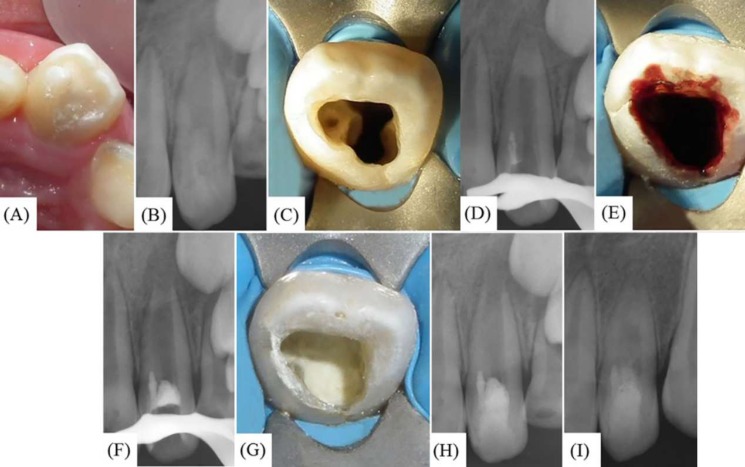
A)* Maxillary left lateral incisor exhibits a cone-shaped morphology; *B)* Pre-operative radiograph showing Oehlers type II DI; *C) *Access cavity depicting two canal orifice entrances; *D)* Radiographic verification of mineral trioxide aggregate (MTA) placement in the invagination; *E)* Blood clot formation; *F)* Radiographic verification of MTA placement in the main canal; *G)* Clinical photograph of white MTA placement in the main canal; *H)* Immediate postoperative radiograph of revascularization technique; *I)* 32 month follow-up with radiographic evidence of notable increase in the thickening of the apical radicular walls, an incrementing in the root length, and resolution of the periapical radiolucency
